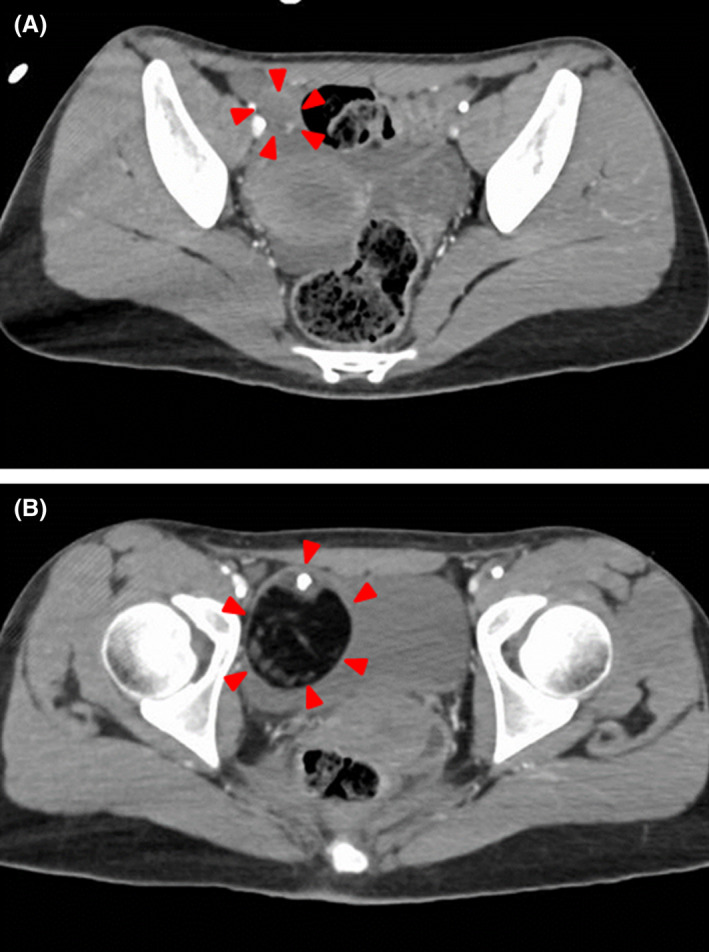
(A) and (B) Pelvic CT showing a tumor with internal calcification in the right ovary (red arrowhead)
